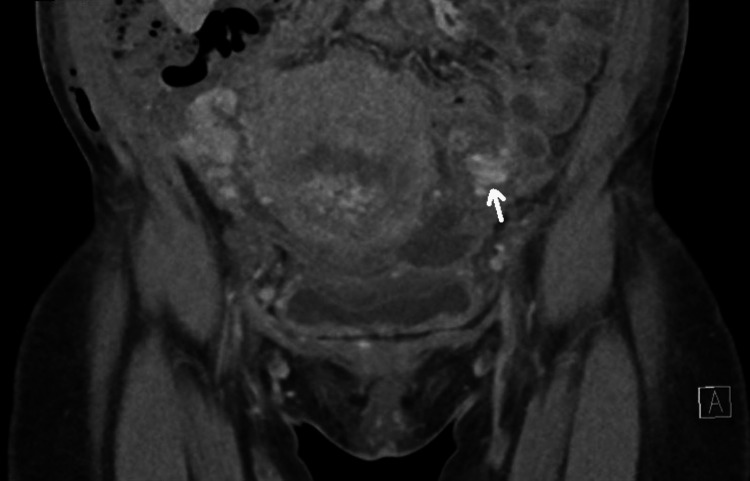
CT scan of the pelvis in Case 3 showing left ovarian vein thrombosis (arrow)
